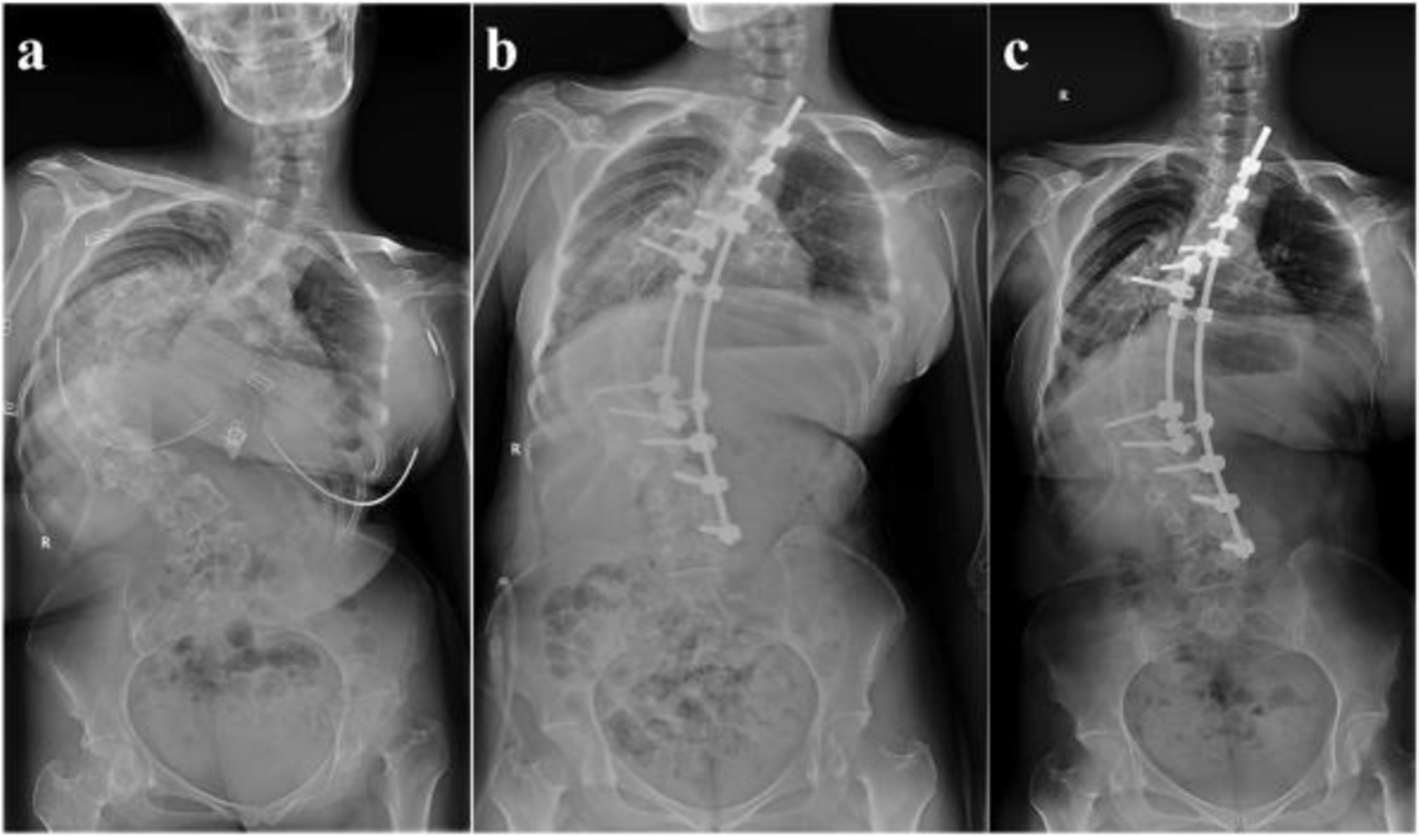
Radiographs obtained in a 30-year-old girl diagnosed idiopathic scoliosis. The patient preoperatively showed proximal, middle, and distal curvatures of 53.40°, 128.40°, and 42.70°, respectively, and RSH − 75.86 mm (a). Immediately after surgery, the patient showed proximal, middle, and distal curvatures of 47.00°, 85.30°, and 23.70°, respectively, and RSH − 43.71 mm (b). The patient showed RSH − 26.72 mm at 2 years’ follow-up (c)
